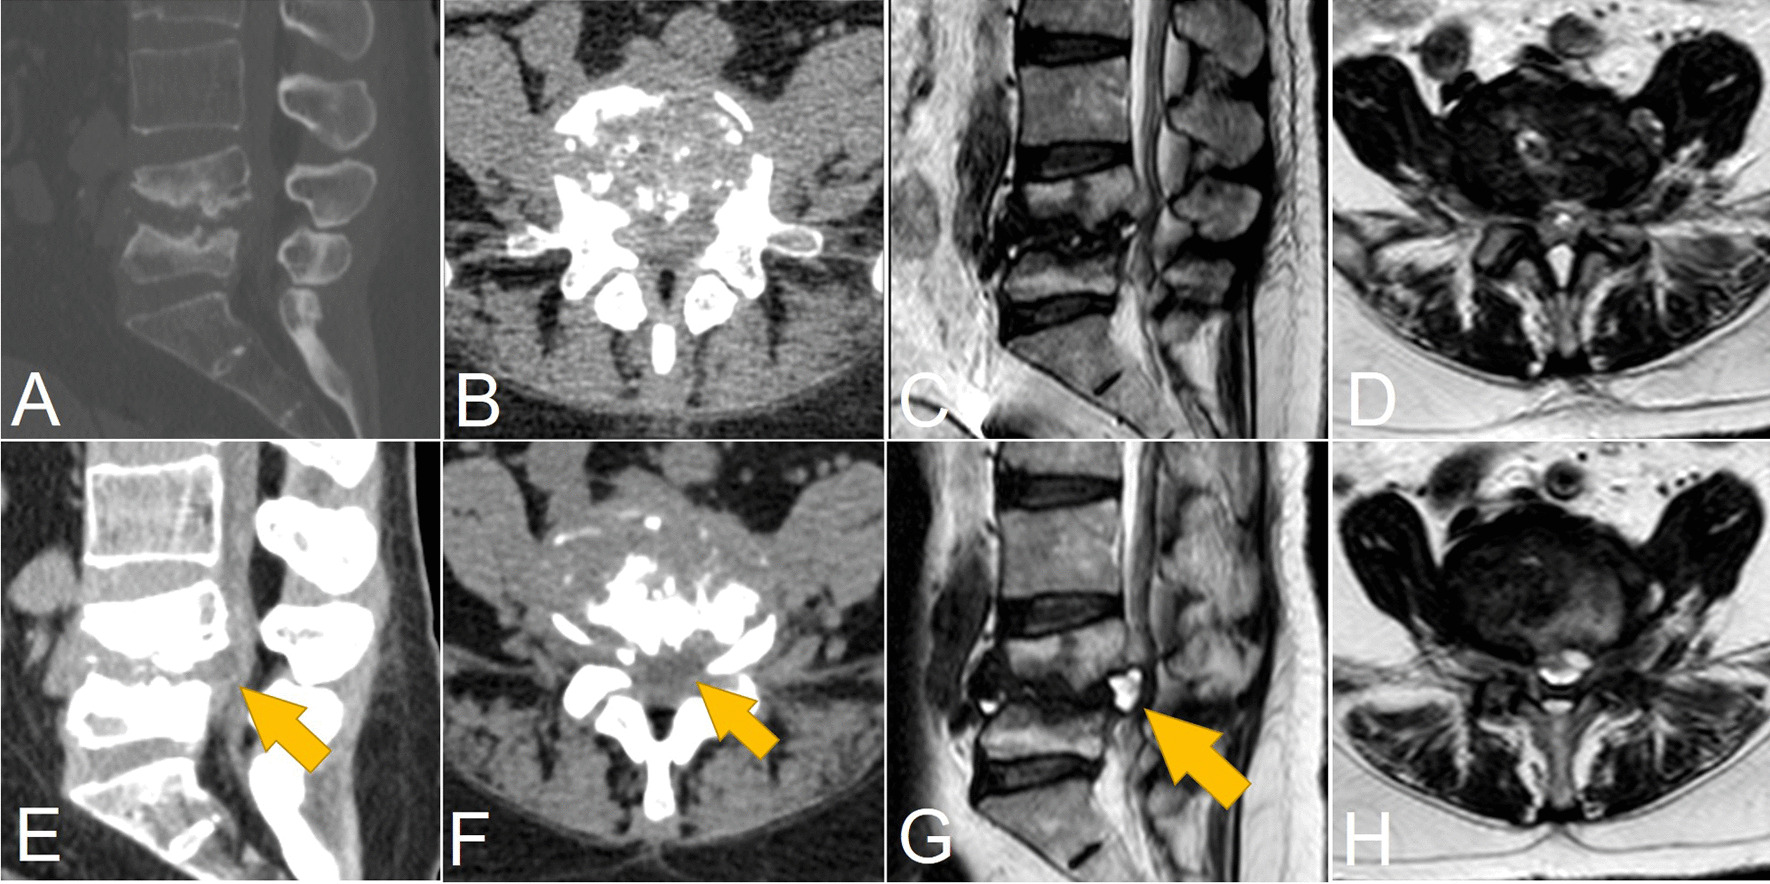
Comparison of CT/MRI images after the second and before the third operation. CT (A, B) and MRI (C, D) images after the second operation. CT (E, F) and MRI (G, H) images before the third operation. Arrow indicated the manifestation of the pseudocyst on CT (E, F) and MRI (G)
